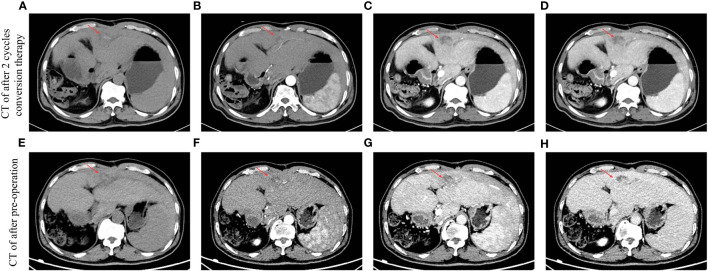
Results of multi-phase CT scans can be evaluated after different periods of conversion therapy. The red arrow indicates an intrahepatic tumor. (A) CT image after two cycles of conversion therapy in the period of non-contrast scan. (B) CT image after two cycles of conversion therapy in the period of the arterial phase. (C) CT image after two cycles of conversion therapy in the period of the portal vein phase. (D) CT image after two cycles of conversion therapy in the period of the delay phase. (E) CT image after preoperation in the period of non-contrast scan. (F) CT image after preoperation in the period of the arterial phase. (G) CT image after preoperation in the period of the portal vein phase. (H) CT image after preoperation in the period of the delay phase.
